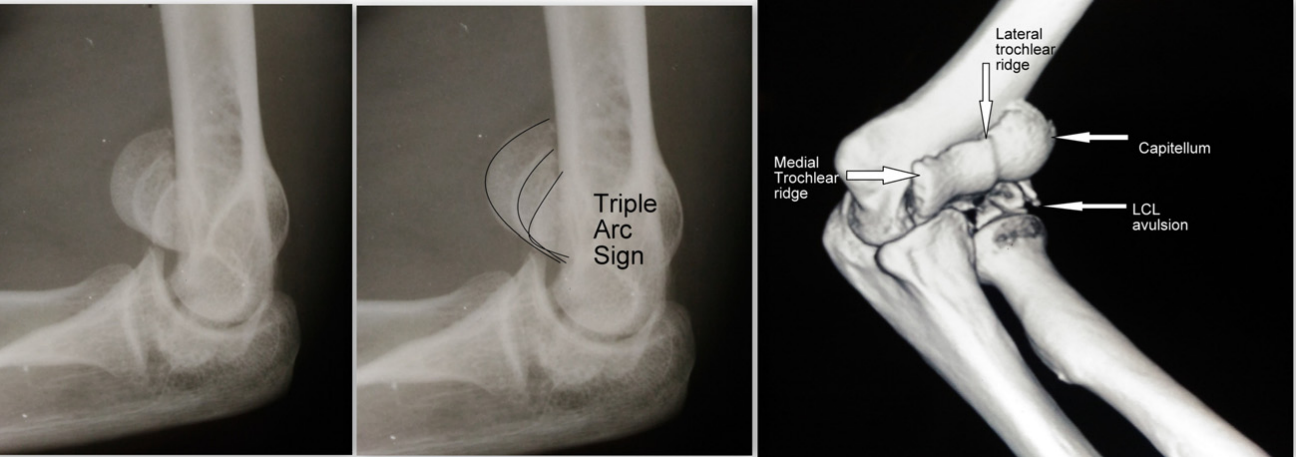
X-rays showing Triple Arc Sign.
